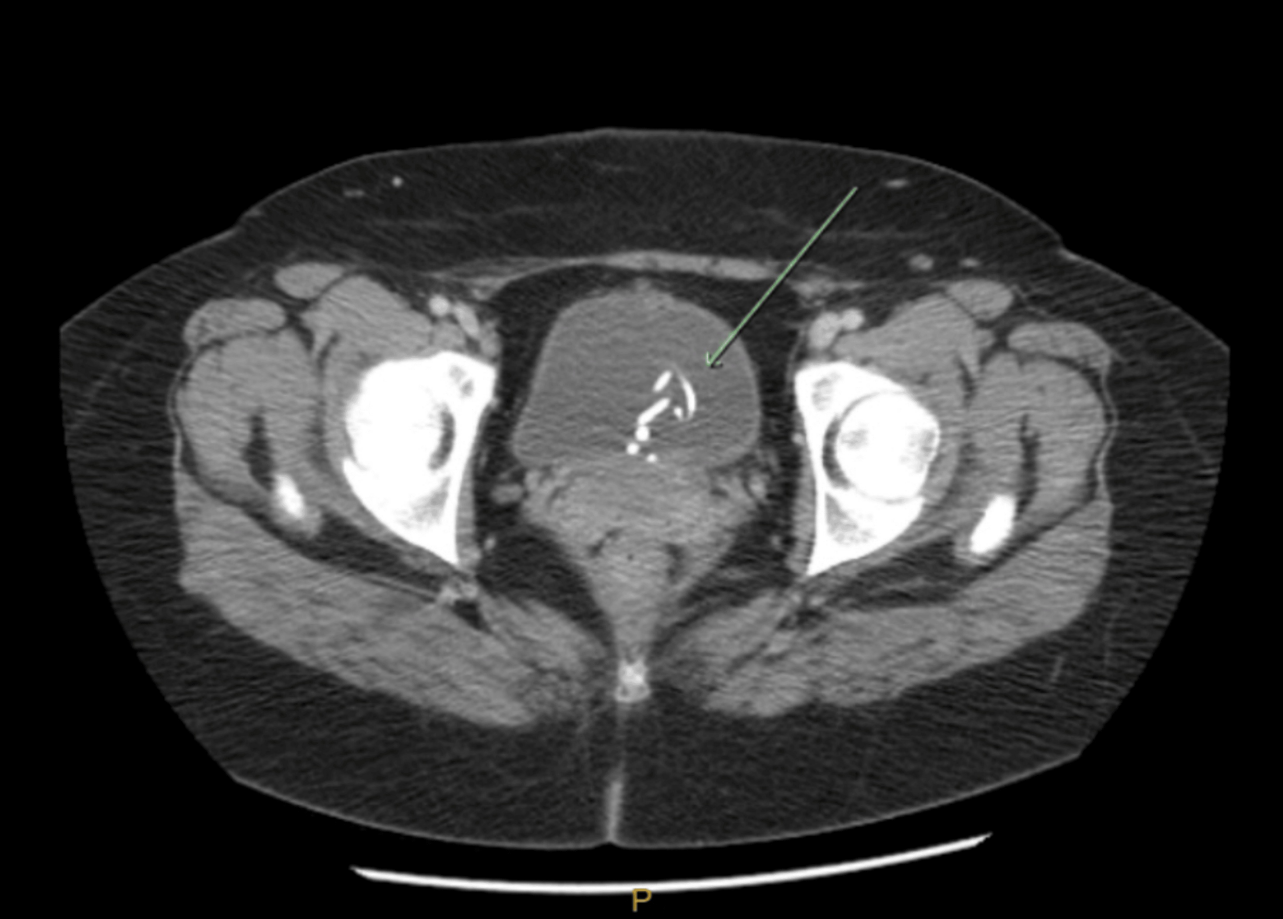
CT scan of the abdomen and pelvis showing a fragmented, curvilinear foreign object in the bladder (green arrow).
